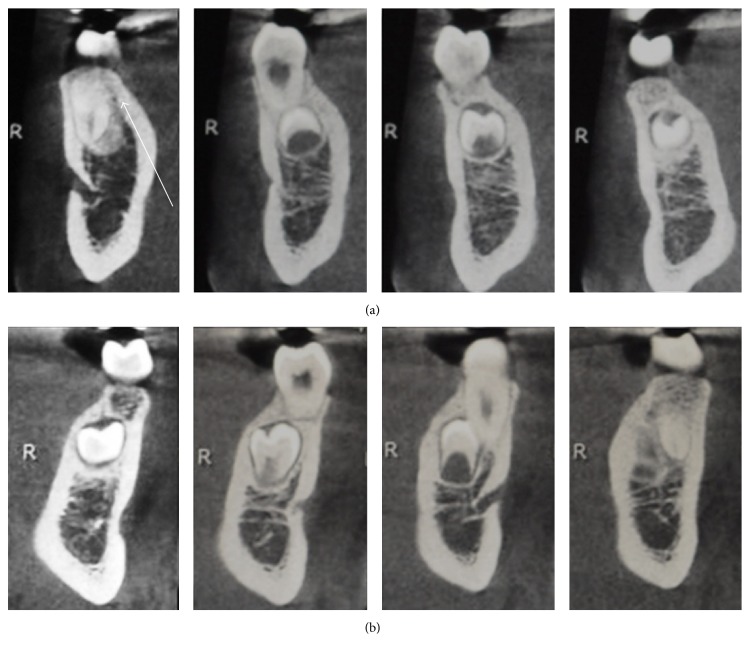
Case 4 CBCT: root resorption of contiguous teeth, indicated by the white arrows ((a) 4.3–4.4 and (b) 3.4–3.5).
